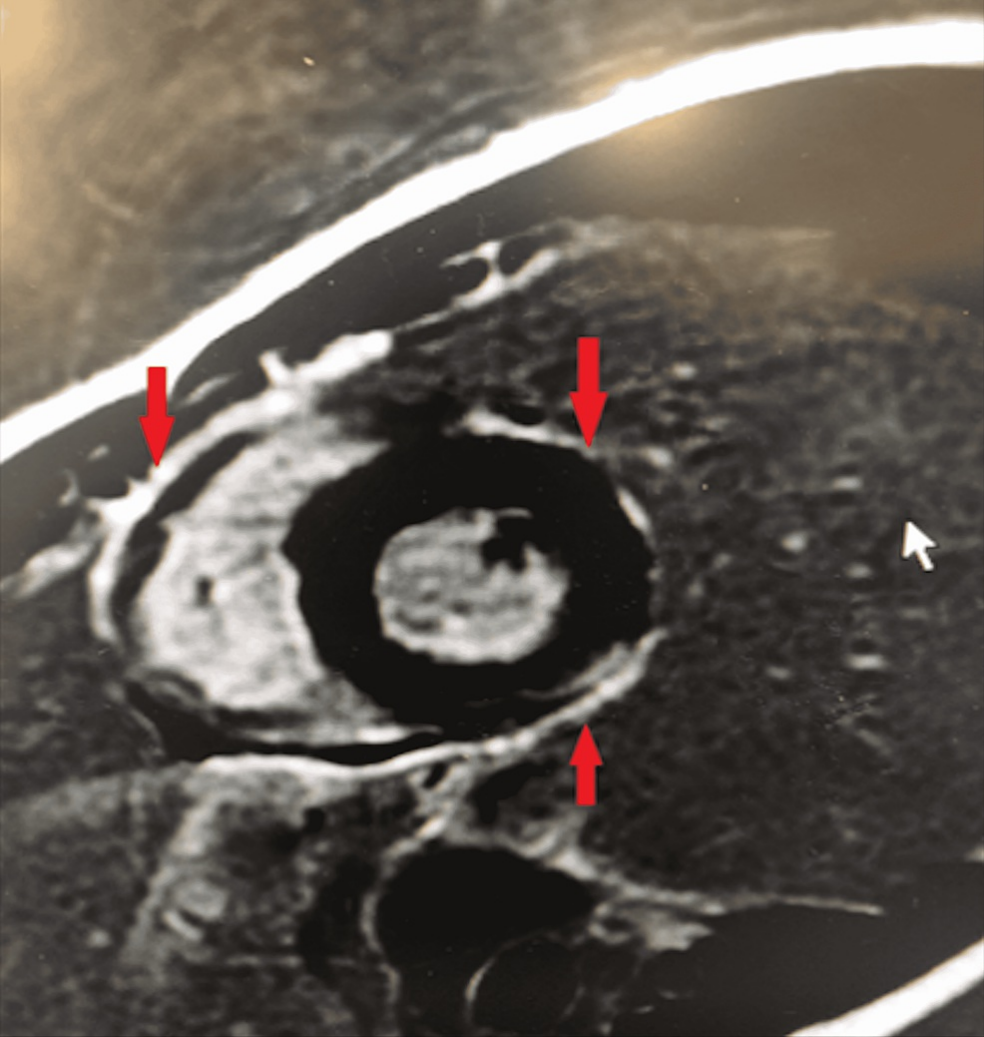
Cardiac Magnetic Resonance Imaging of Patient 1 Sort axis post-contrast image depicting pericardial enhancement (red arrows) adjacent to the right ventricle free wall and the lateral left ventricle wall.
